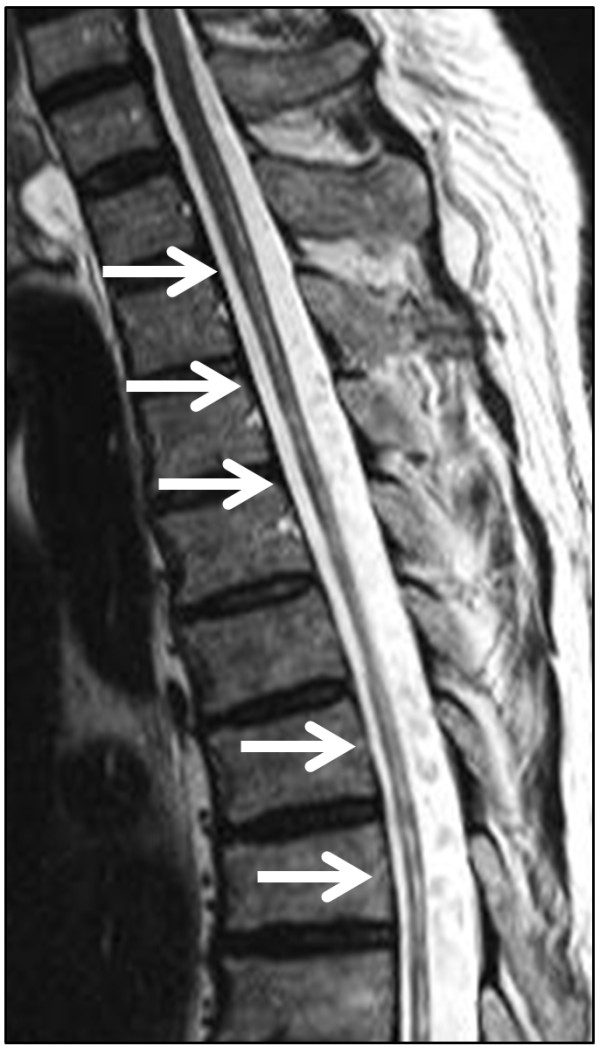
Spinal cord magnetic resonance imaging (T2-weighted imaging from C4 to Th6) one year after the start of combination treatment with rituxan and cyclophosphamide depicting longitudinally extensive spinal cord lesions (see arrows) with significant cord atrophy.
